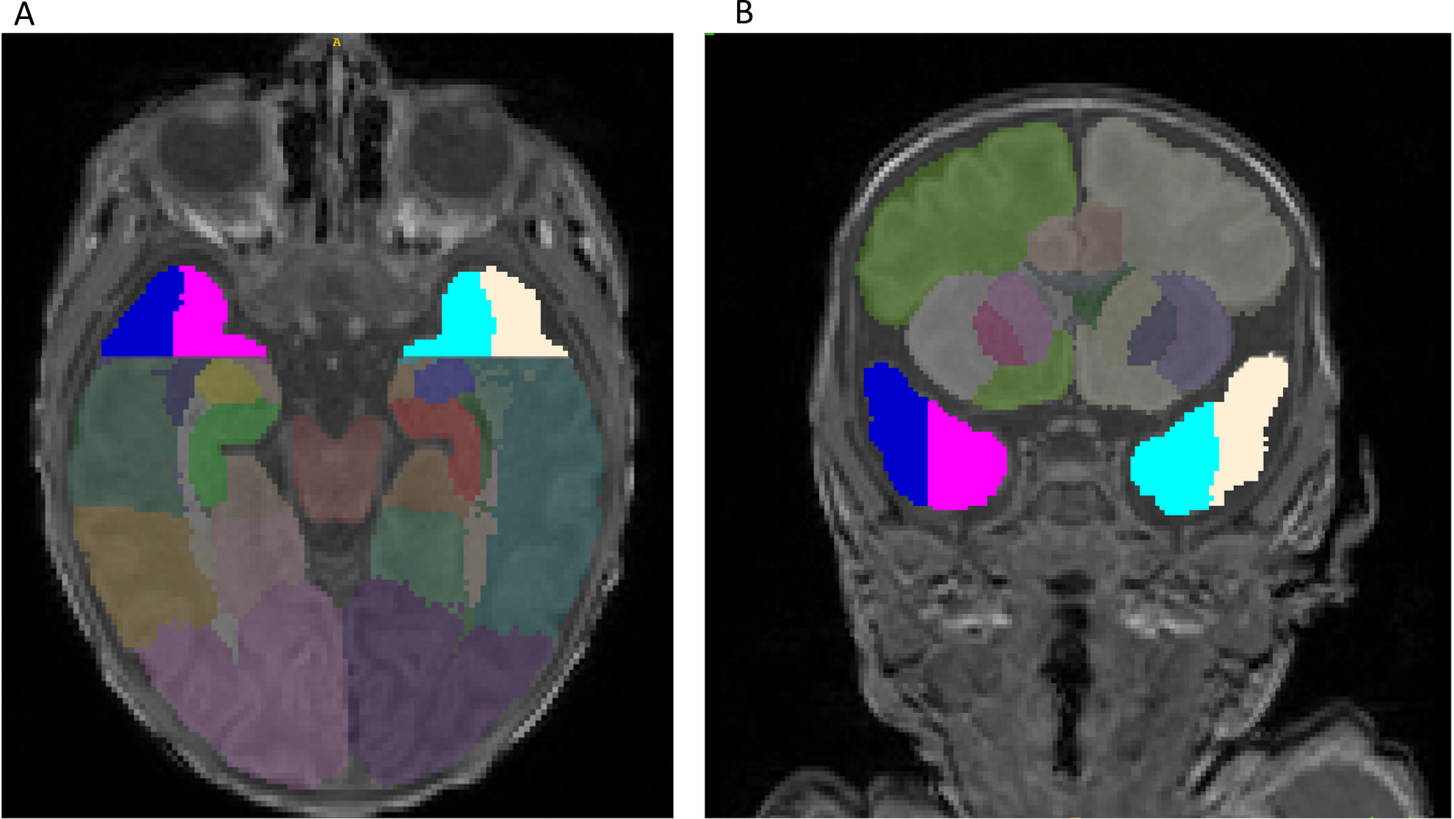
Brain MRI segmentation: A. axial and B. coronal view T1 images. Note. Colors highlight anterior temporal lobe (ATL) lateral part left (ATL-LPL, yellow) and right (ATL-LPR, dark blue) as well as ATL medial part left (ATL-M;PL, light blue) and right (ATL-MPR, pink).
